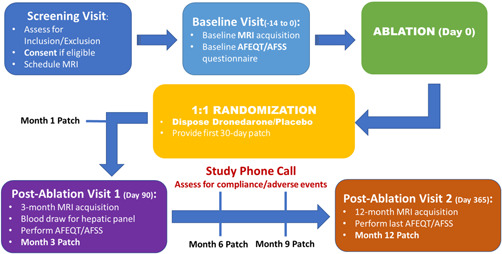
Follow‐up design for the EDORA trial. AFEQT, atrial fibrillation effect on QualiTy‐of‐life, AFSS, Atrial Fibrillation Severity Scale; MRI, magnetic resonance imaging
